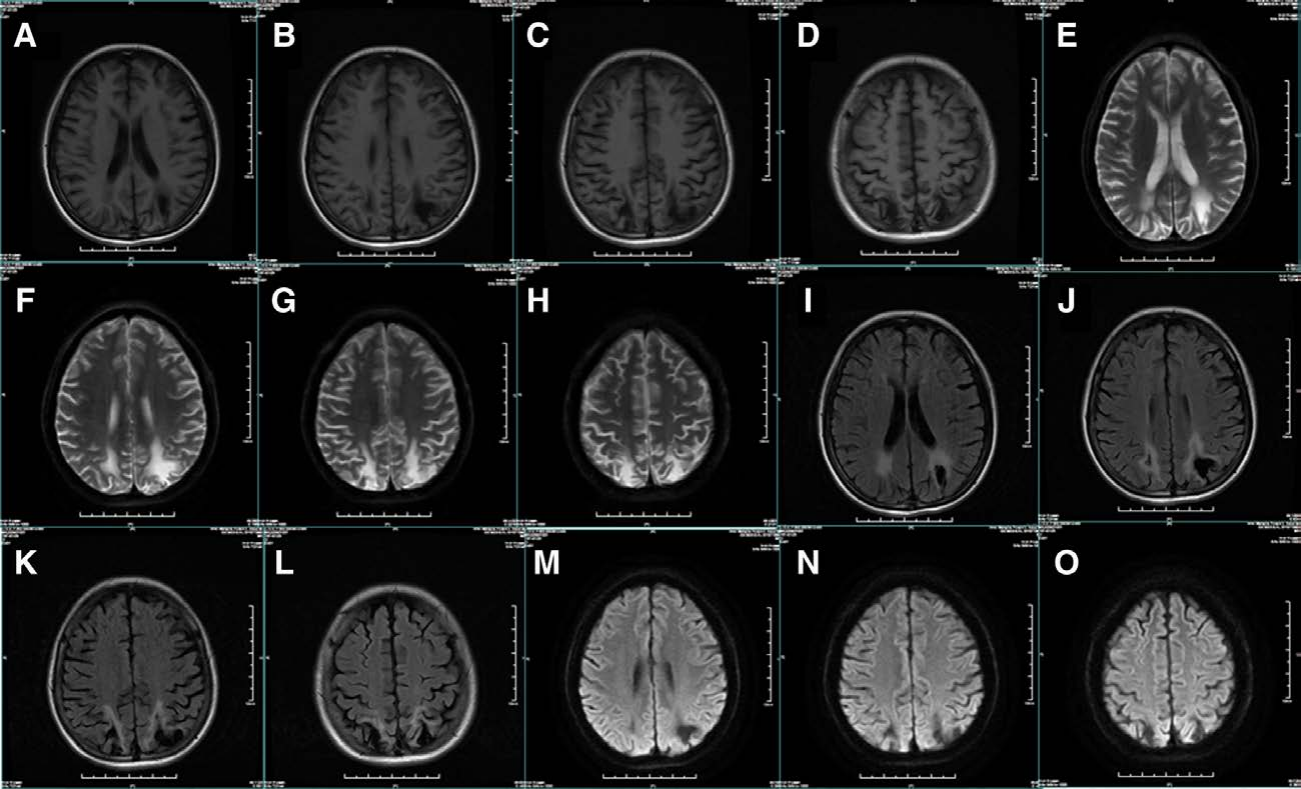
Magnetic resonance imaging of the patient.
